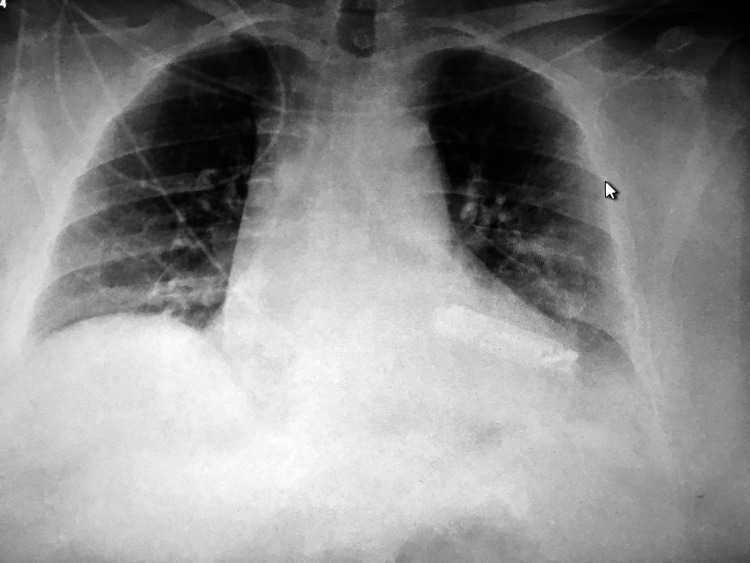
Follow-up chest X-ray showing significant improvement with resolution of the right upper lobe infiltrate and tracheal deviation
